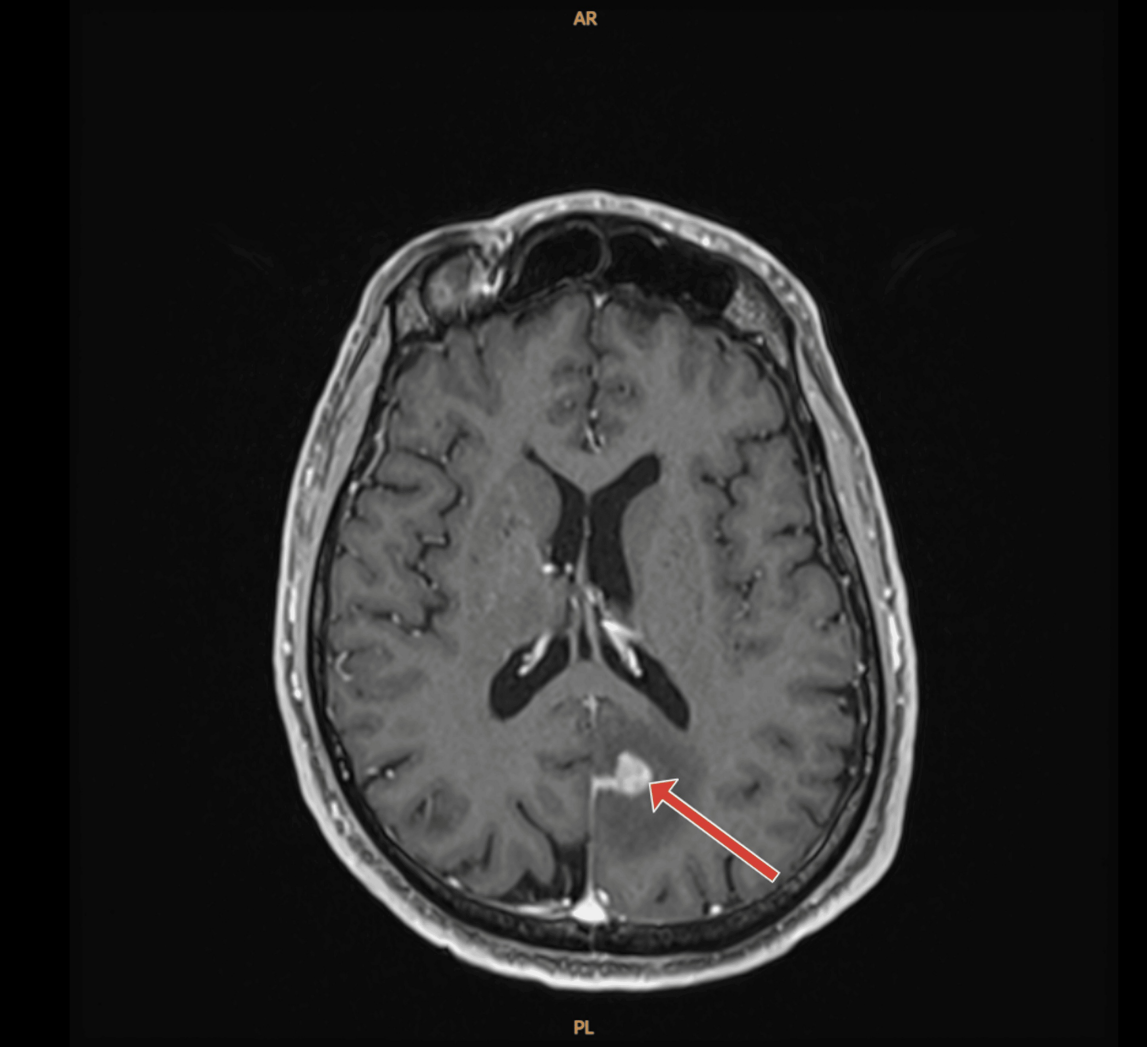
Post-contrast axial MRI T1-weighted of the brain, with red arrow pointing to marked mass like pial enhancement in the left parieto-occipital area concerning for leptomeningeal carcinomatosis.
